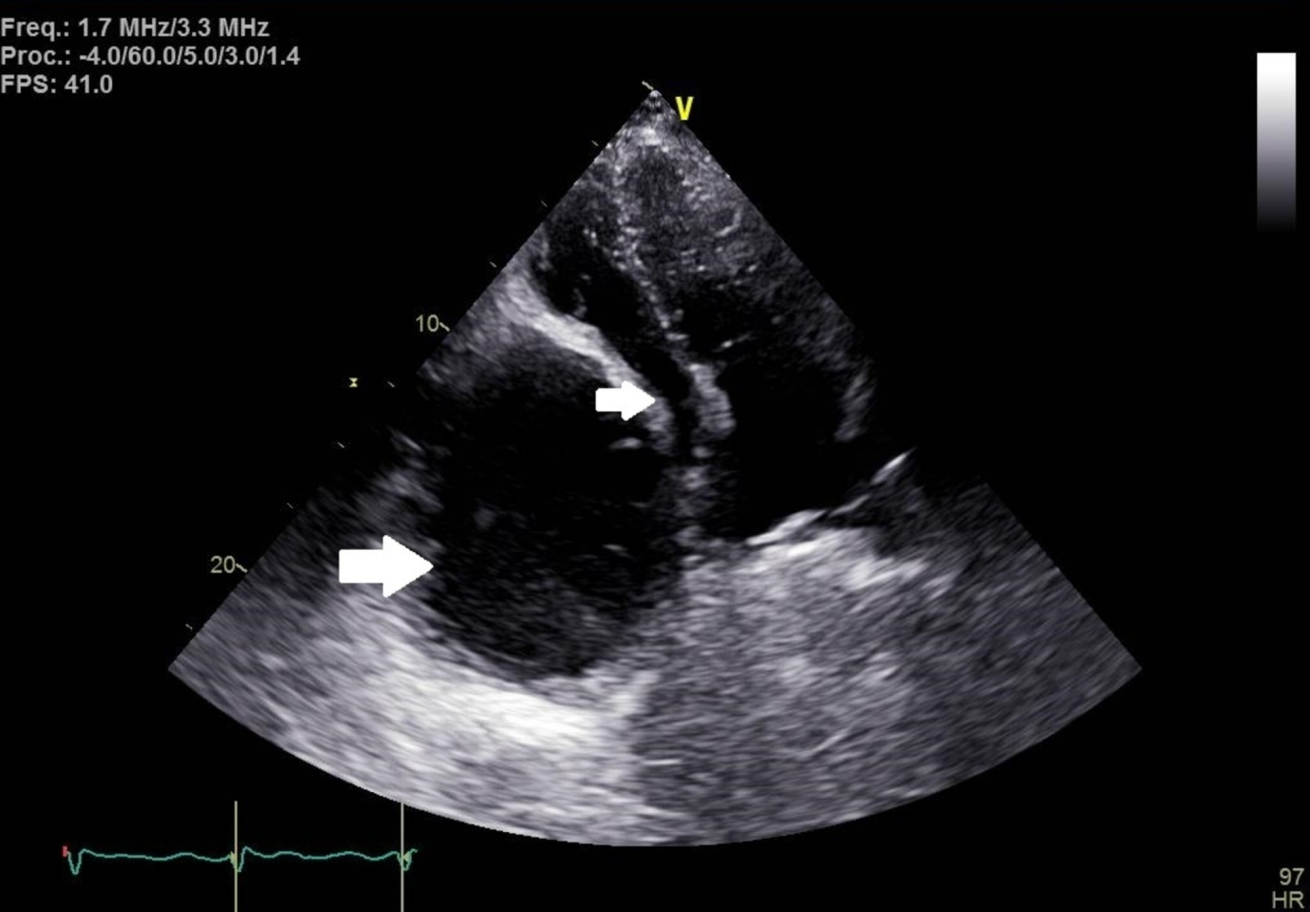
Transthoracic echocardiograph with apical four-chamber view, with the upper arrow indicating the right atrium and the lower arrow indicating liver cyst compressing the right atrium
